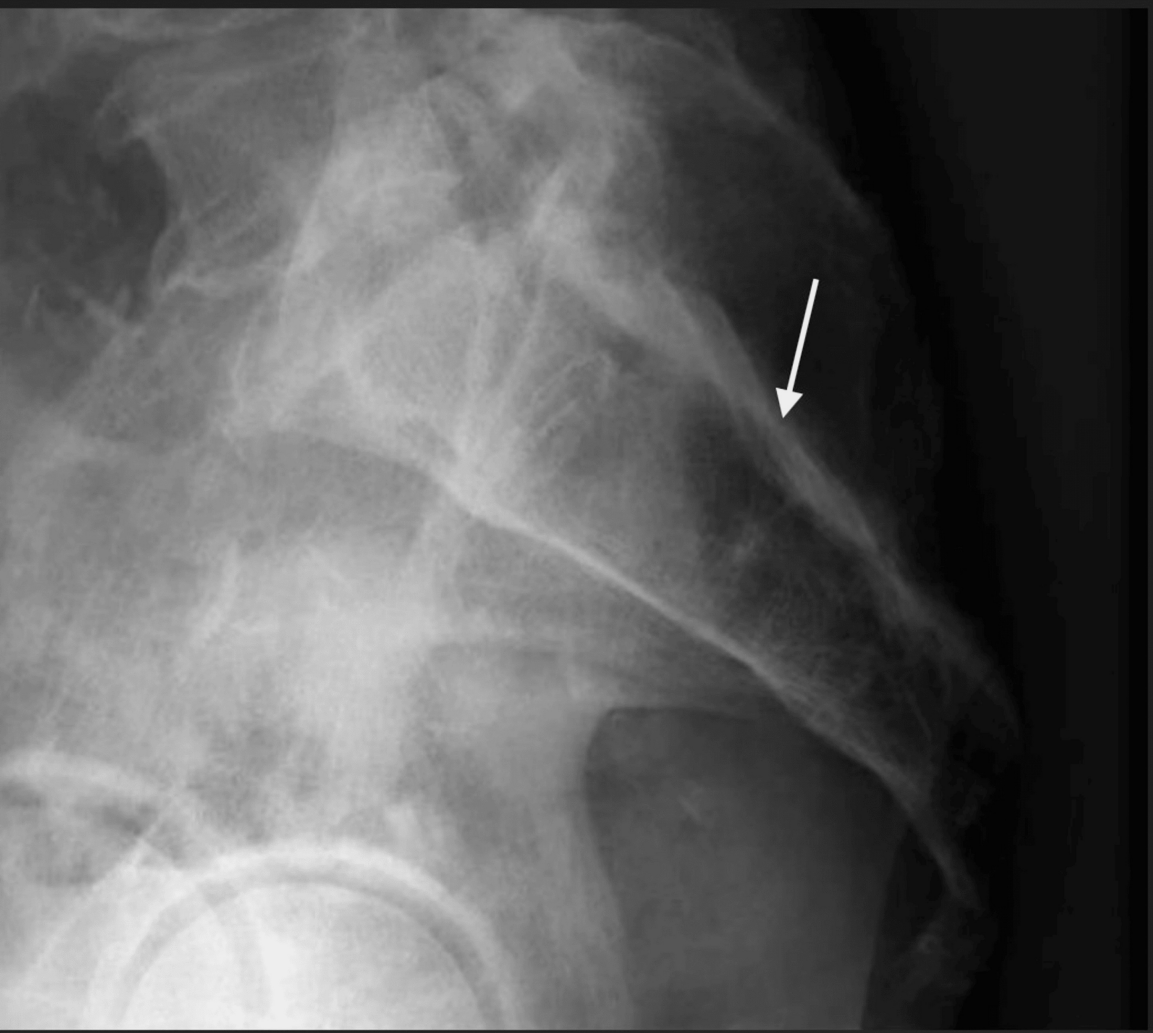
Plain film X-ray of sacrum Plain film X-ray lateral view of the sacrum showing osteomyelitis of the distal sacrum/coccyx (white arrow)
